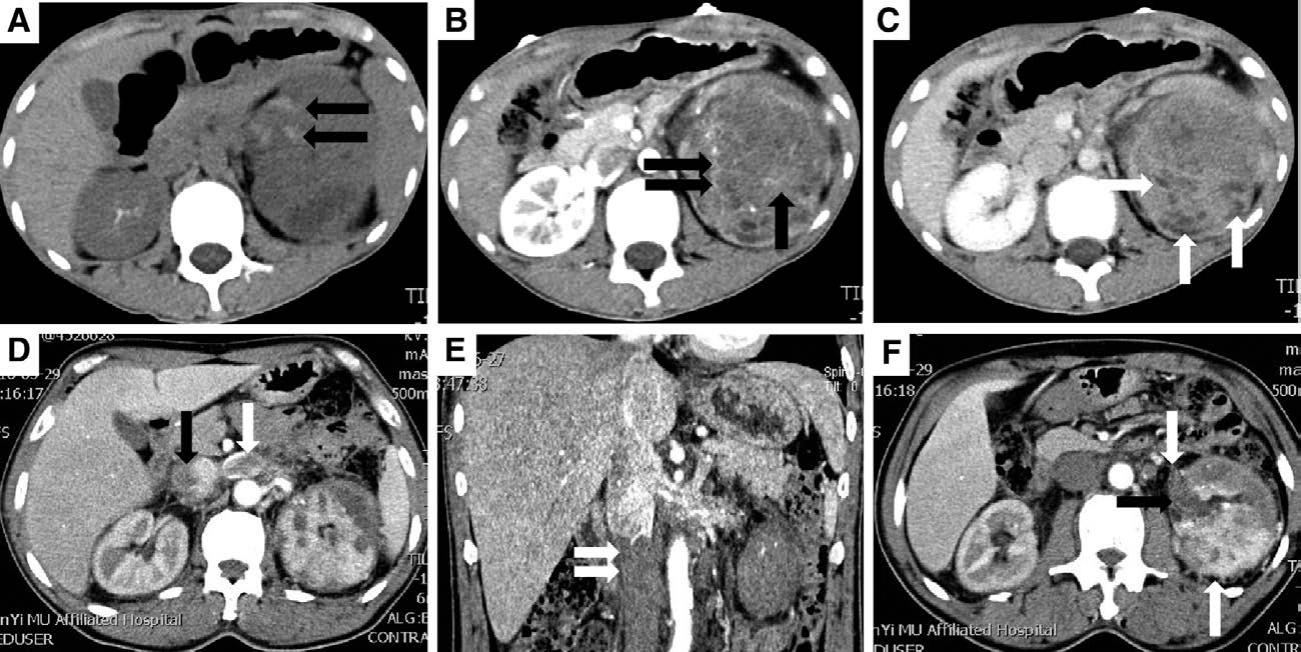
(A). A 12-year-old male patient was hospitalized due to abdominal pain and accidental hematuria, CT revealed a large soft tissue mass with inhomogeneous density in the left renal region, and nodules and patchy high-density hemorrhagic shadows (black arrows); (B). In the cortical stage of contrast-enhanced CT, the lesion was slightly inhomogeneous in enhancement, and the septum-like enhancement shadow was visible in it (black arrows); (C). Contrast-enhanced CT in the nephrographic phase of the lesion continued to non-uniform enhancement, the tumor surrounding a number of small nodular cystic lesions with no enhancement (black arrows); (D). An 18-year-old woman was admitted to hospital due to abdominal pain. Contrast-enhanced CT showed an inhomogeneous density soft tissue mass shadow in the left kidney, filling defects in the renal vein (white arrow) and inferior vena cava (black arrow), suggesting tumor thrombosis; (E). The coronal position of multiplanar reconstruction clearly shows the thrombus of inferior vena cava (white arrows); (F). Contrast enhanced CT showed tumor invasion of renal sinus (black arrow) and perirenal fat (white arrows). CT = computed tomography.
